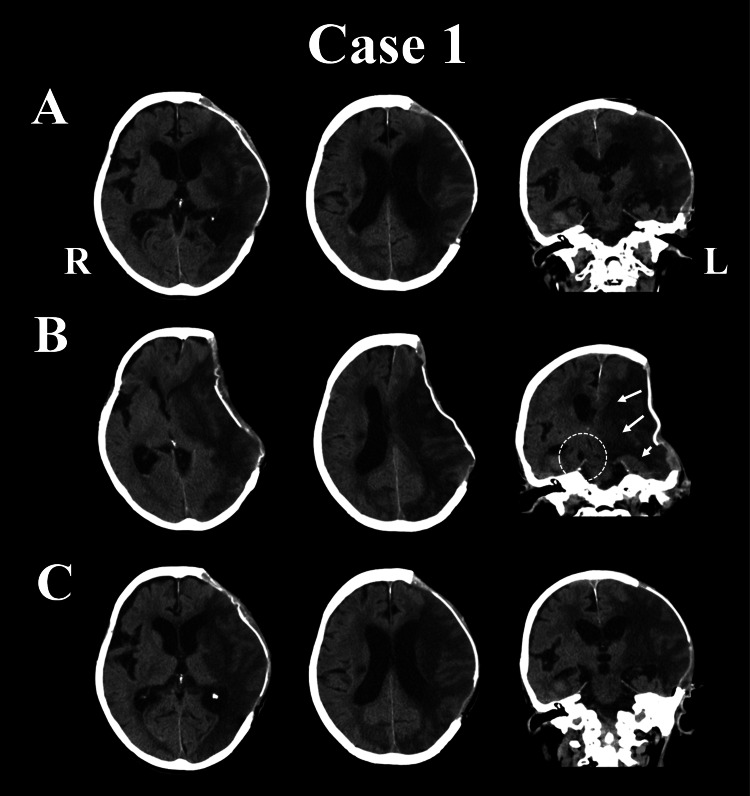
CT findings in Case 1. (A) Mild ventriculomegaly is apparent. The left hemisphere is protruding from the cranium, and the skin at the site of the bone defect is bulging. (B) There is a remarkable sinking of the skin at the site of the bone defect, causing a midline shift to the side contralateral to the bone defect. Because the bone defect has been compressed inward and downward by atmospheric pressure (white arrows), the midbrain has shifted to the right side, resulting in the Kernohan notch phenomenon (white circle). (C) The ventricle has enlarged, the midline shift has resolved, and the compression of the midbrain by the cerebellar tent is less marked. CT: computed tomography
